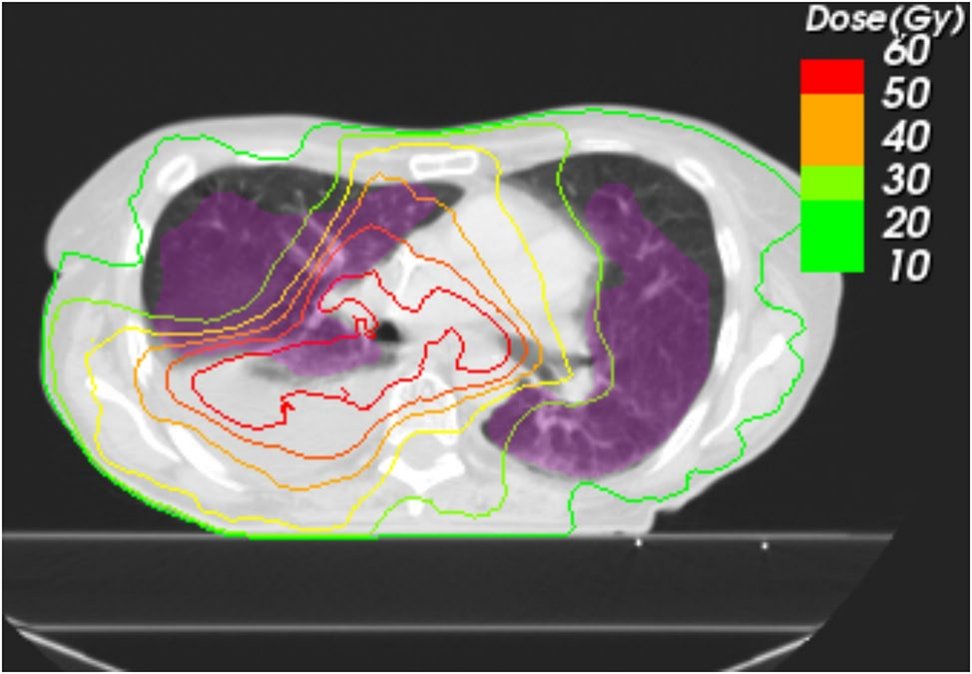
Axial Computed Tomography (CT) scan with EQD2 isodose lines and the pneumonitis contours matched in color-washed magenta. The majority of the pneumonitis volume is located outside the high dose region
